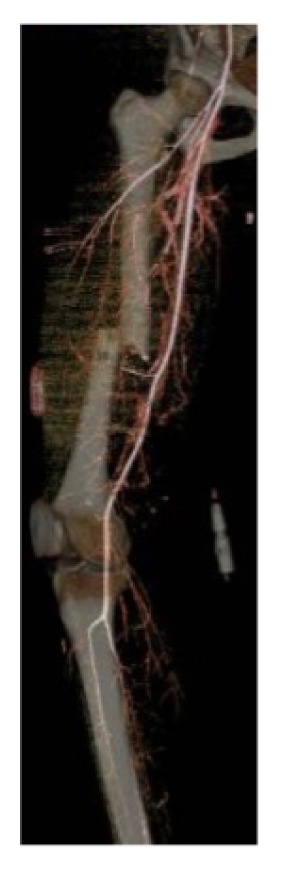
3D reconstruction of CTA angiogram performed preoperatively. No arterial injury was identified. Note the transverse middle third femoral shaft fracture.
